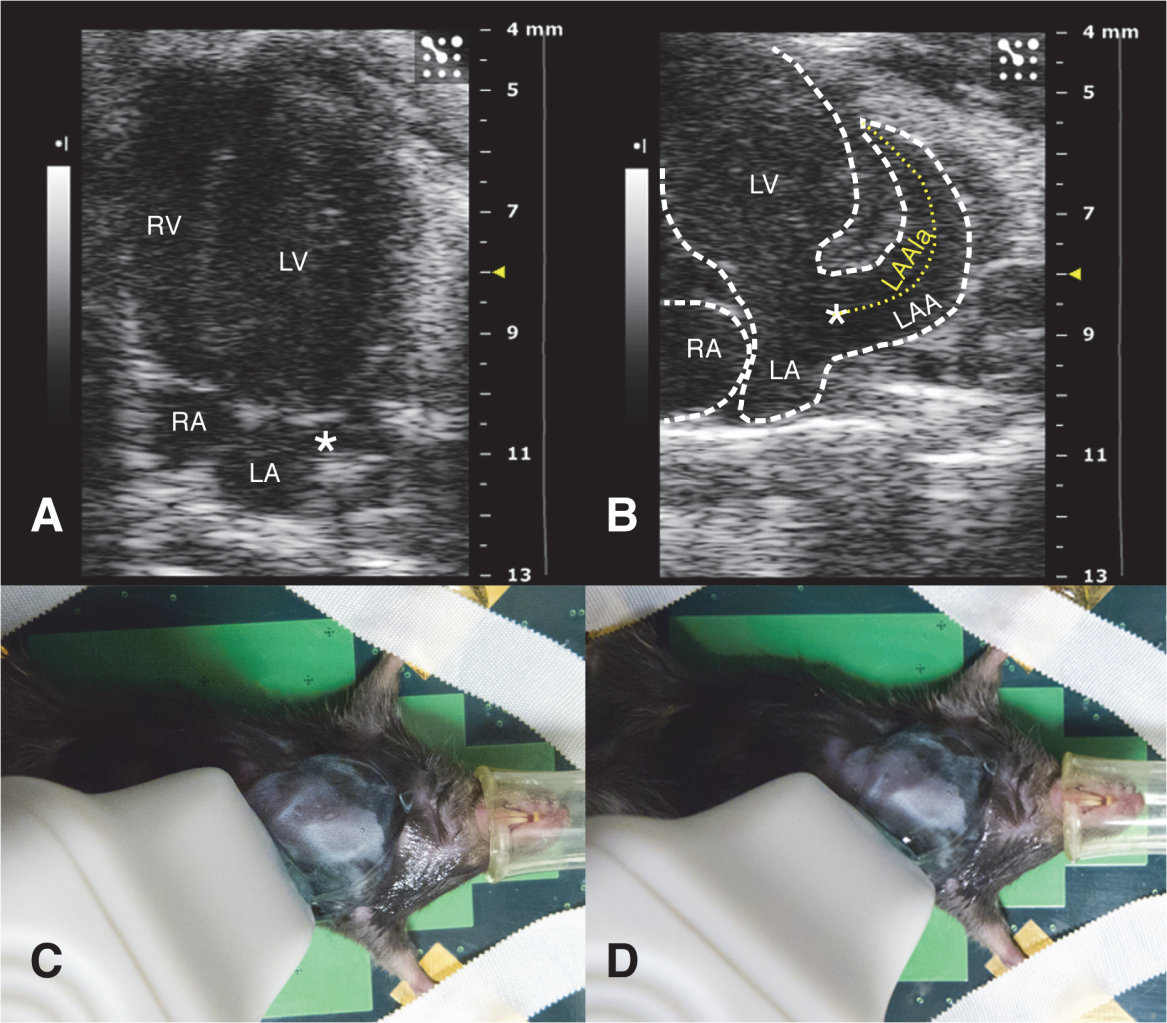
Standard and modified apical 4-chamber views. A) Standard apical 4-chamber view, optimized for the left atrium. B) Modified apical 4-chamber view to visualise the LAA and duct (*); the dotted line shows the measurement of the long axis C) Transducer position to obtain A. D) Transducer rotated counter-clockwise starting from C to obtain B. *: LA-LAA duct; LA: left atrium; LAA: left atrial appendage; LAAla: LAA long axis; LV: left ventricle; RA: right atrium; LV: left ventricle; RV: right ventricle.
